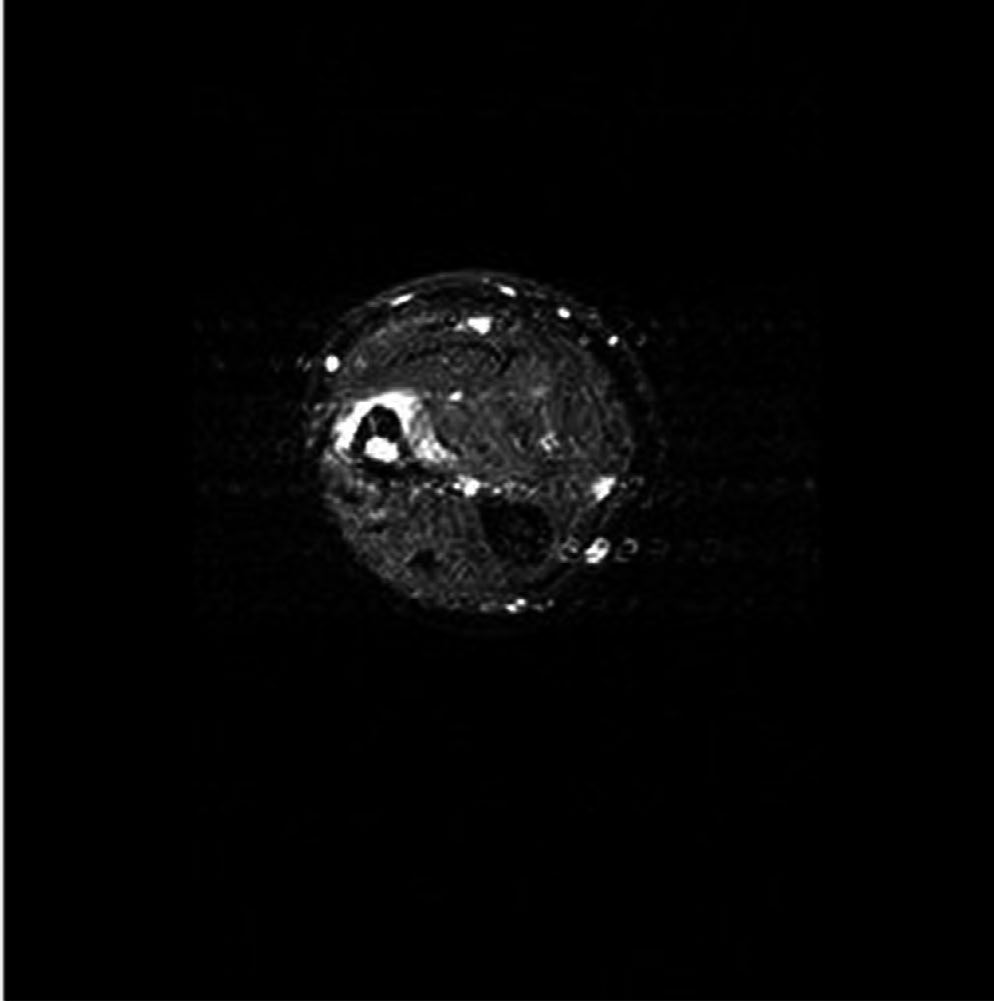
MRI without contrast, T2, Axial view
